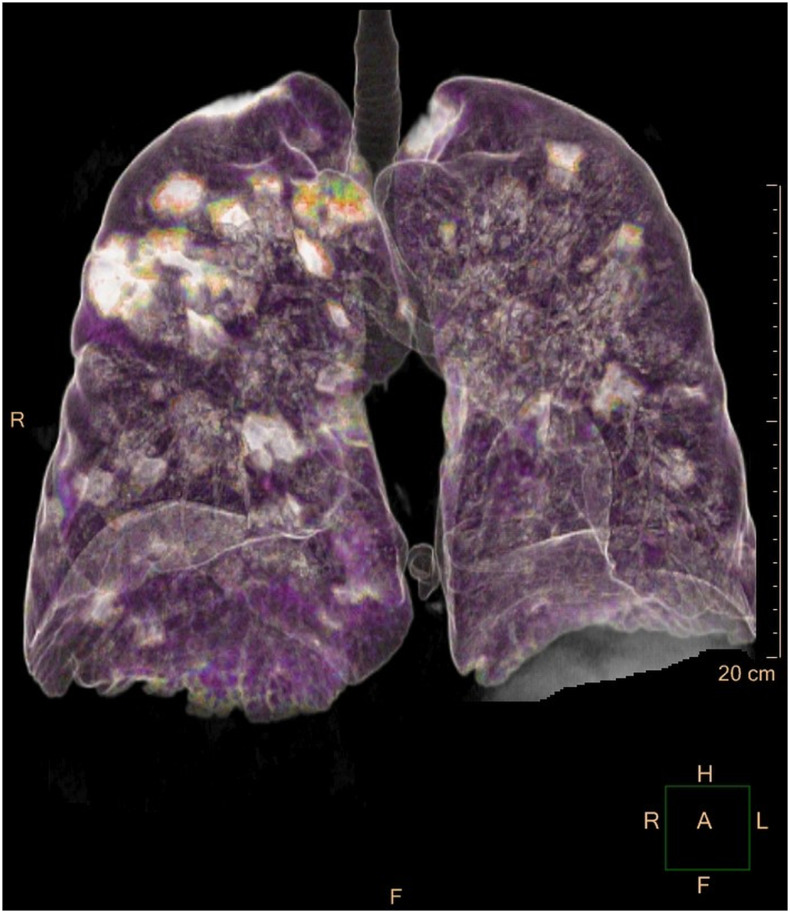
Volume-rendered three-dimensional reconstruction chest CT image shows the presence of bilateral opacities, with prevalent peribronchovascular distribution and sparing of the peripheral lung zones.
